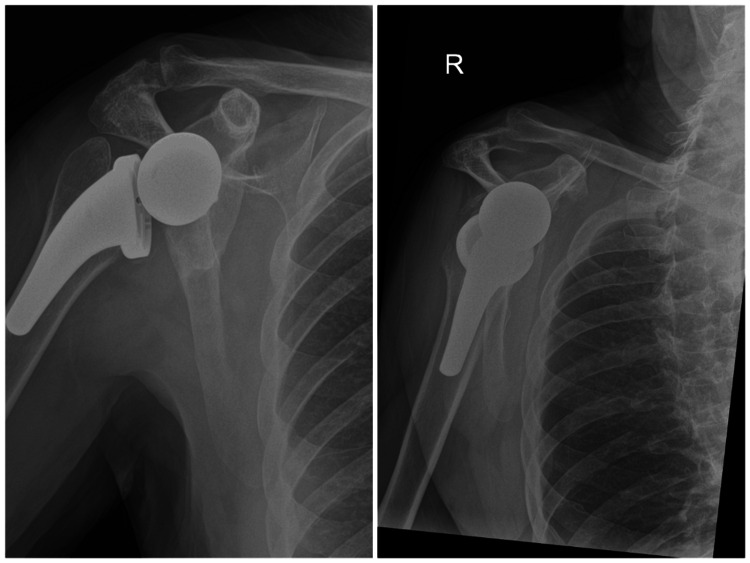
Four-year post-operative radiographs demonstrating maintained implant position with no radiographic evidence of loosening, migration or failure.
